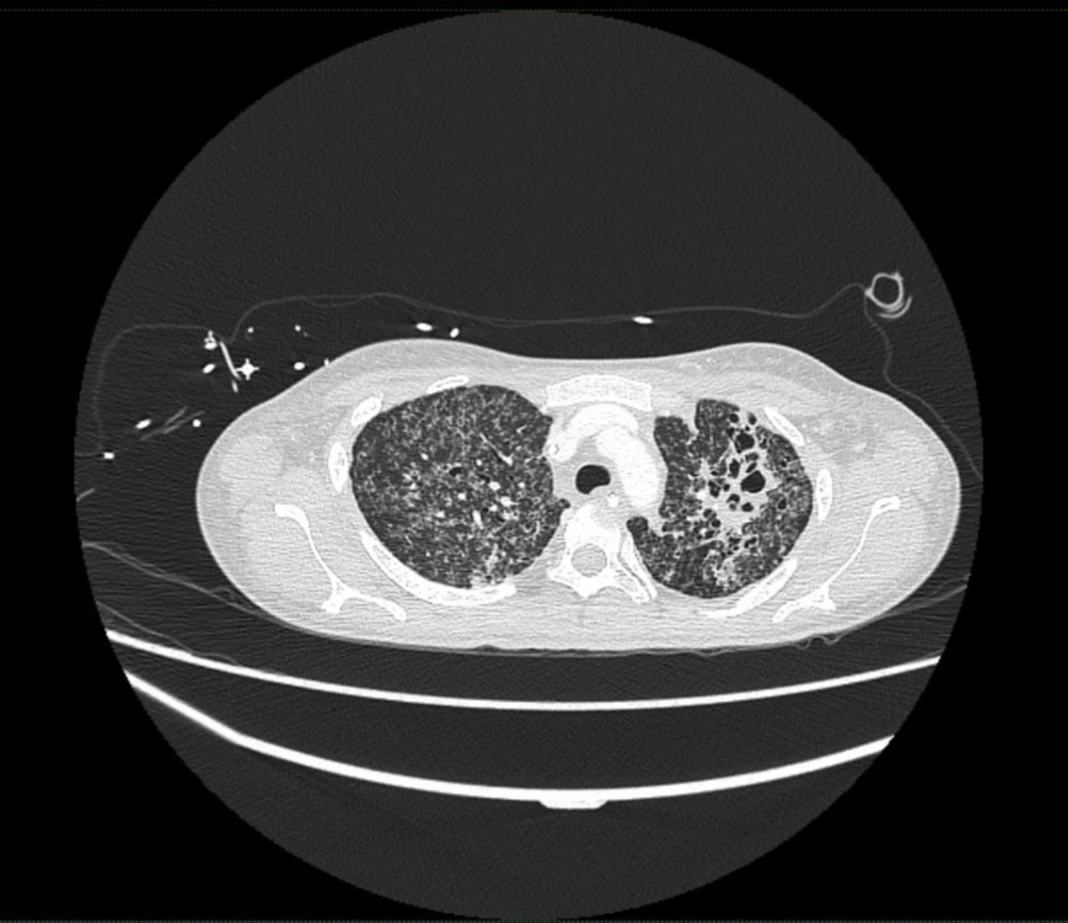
Chest Computed Tomography Showing Cavitation of the Upper Lobes of the Lungs
